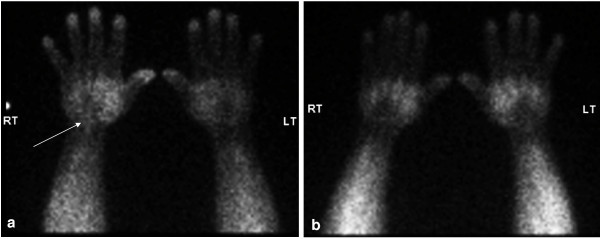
Thallium-201 scintigraphy at a) 30 minutes demonstrates focal uptake in the right wrist (arrow), and b) at four hours, wash-out in this area had occurred.
